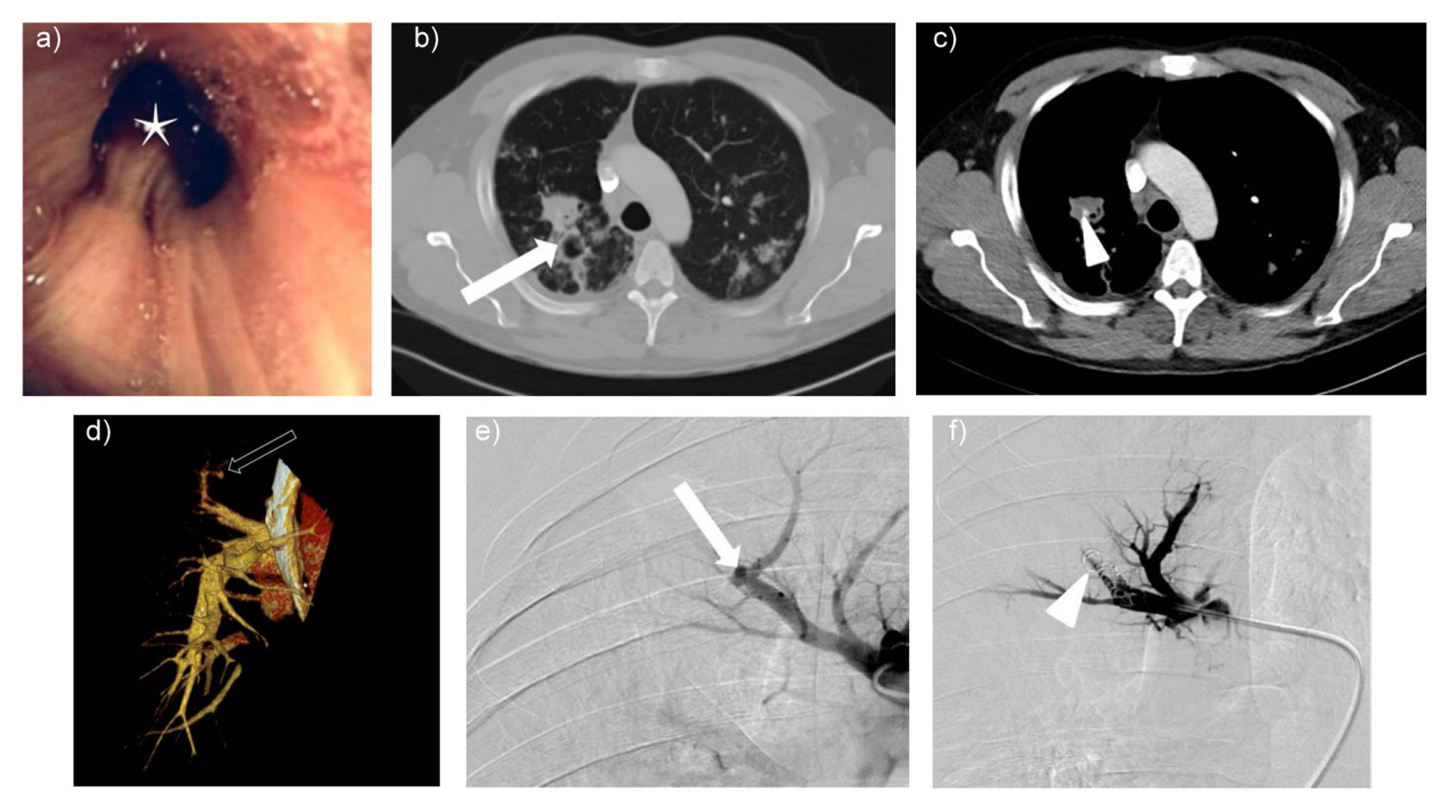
a) Bronchoscopy showed a clot in the right upper lobe bronchus. b), c) and d) CT angiography showed consolidations and a peribronchial nodule at the level of the apical segment of the right upper lobe (b, white arrow) and a 7 mm contrast-enhancing focus (c, arrow head) originating from the right pulmonary artery, as visualised on volume rendering reconstruction (d, black arrow), suggesting an aneurysm of the pulmonary artery. e) and f) Digital subtraction angiography after pulmonary artery catheterism showed the aneurysm in the right upper lobe (e, arrow). Selective embolization was performed with microcoils (f, arrowhead).
